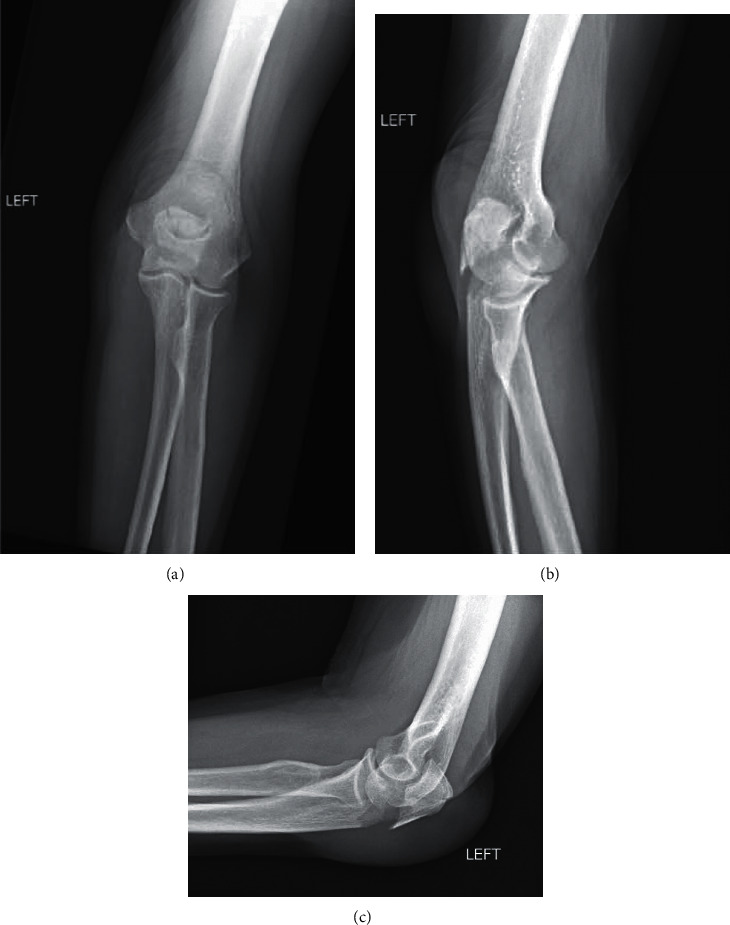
Preoperative AP (a), oblique (b), and lateral (c) radiographs demonstrating a displaced and comminuted left olecranon fracture.
